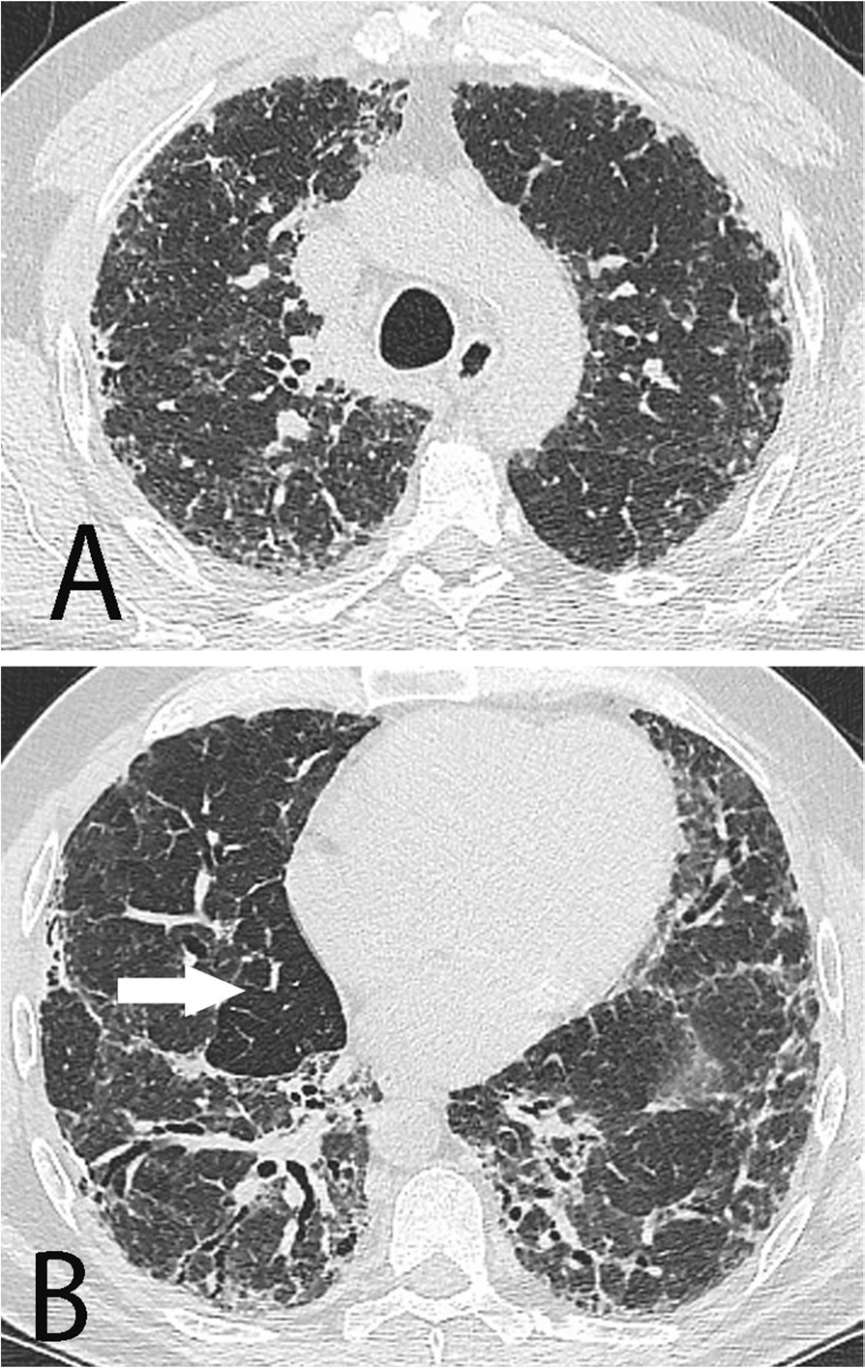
HRCT of the chest in the axial plane shows reticulation and traction bronchiectasis consistent with findings of moderate-severity interstitial fibrosis. There is no honeycombing. Patchy ground-glass opacities are present along with mosaic perfusion pattern with lobular areas of air-trapping (arrow, image B). The disease involve all pulmonary lobes, including upper lung zones (image A) but is more severe in the lower lobes (image B)
